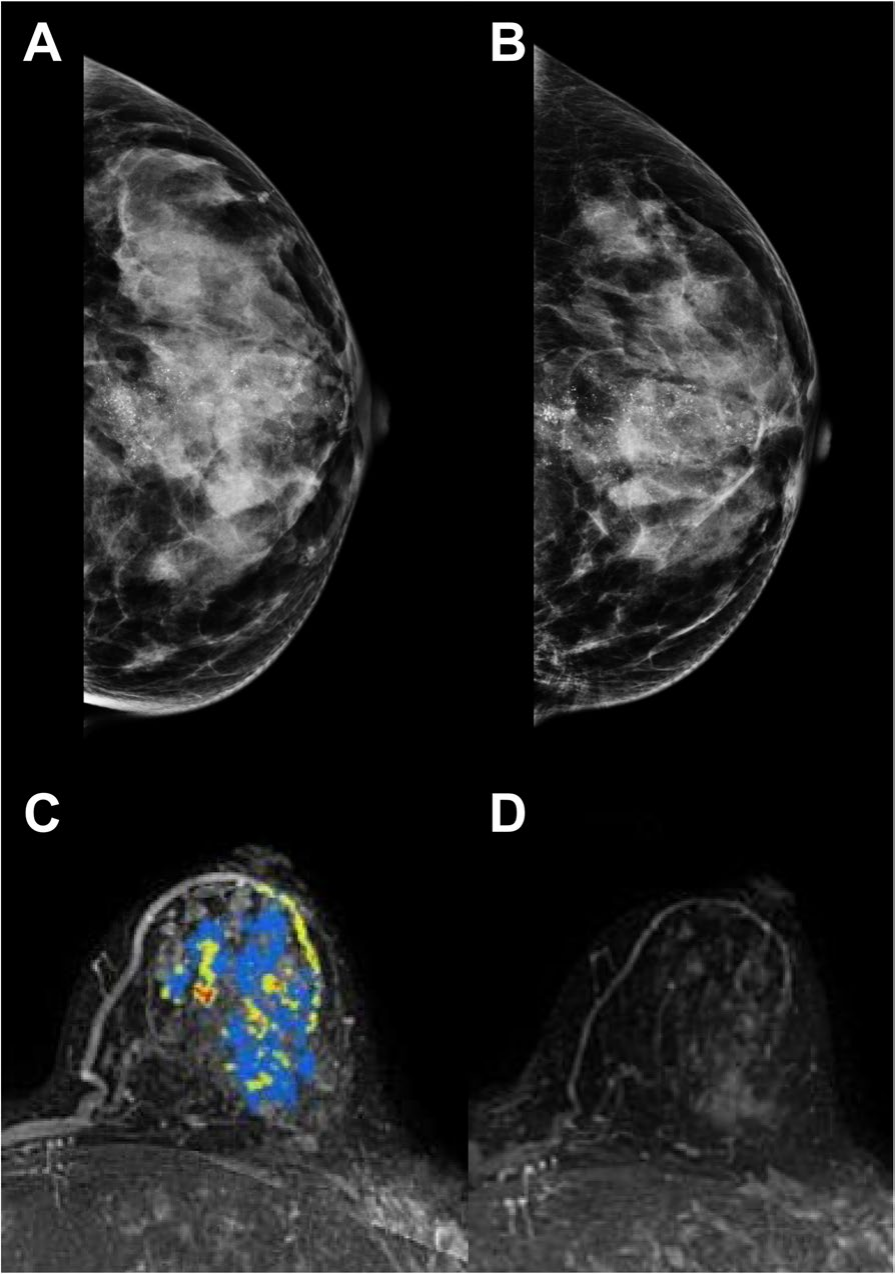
A 52-year-old woman with 44-mm HR^+^/HER2^−^ subtype in the lower central quadrant of the left breast. Before NAC, A a CC image of mammography revealed a 62-mm extent of segmental distribution of microcalcifications. After NAC, B the size, mass, and extent of microcalcifications did not decrease on mammography. On C a computer-aided detection (CAD) image from MRI, a 44-mm, irregularly shaped, non-mass-like enhancement was noted. After NAC, D the CAD image from MRI revealed that the enhancement had decreased to a 35-mm extent. She underwent total mastectomy, and the pathology showed a residual DCIS of 42 mm
